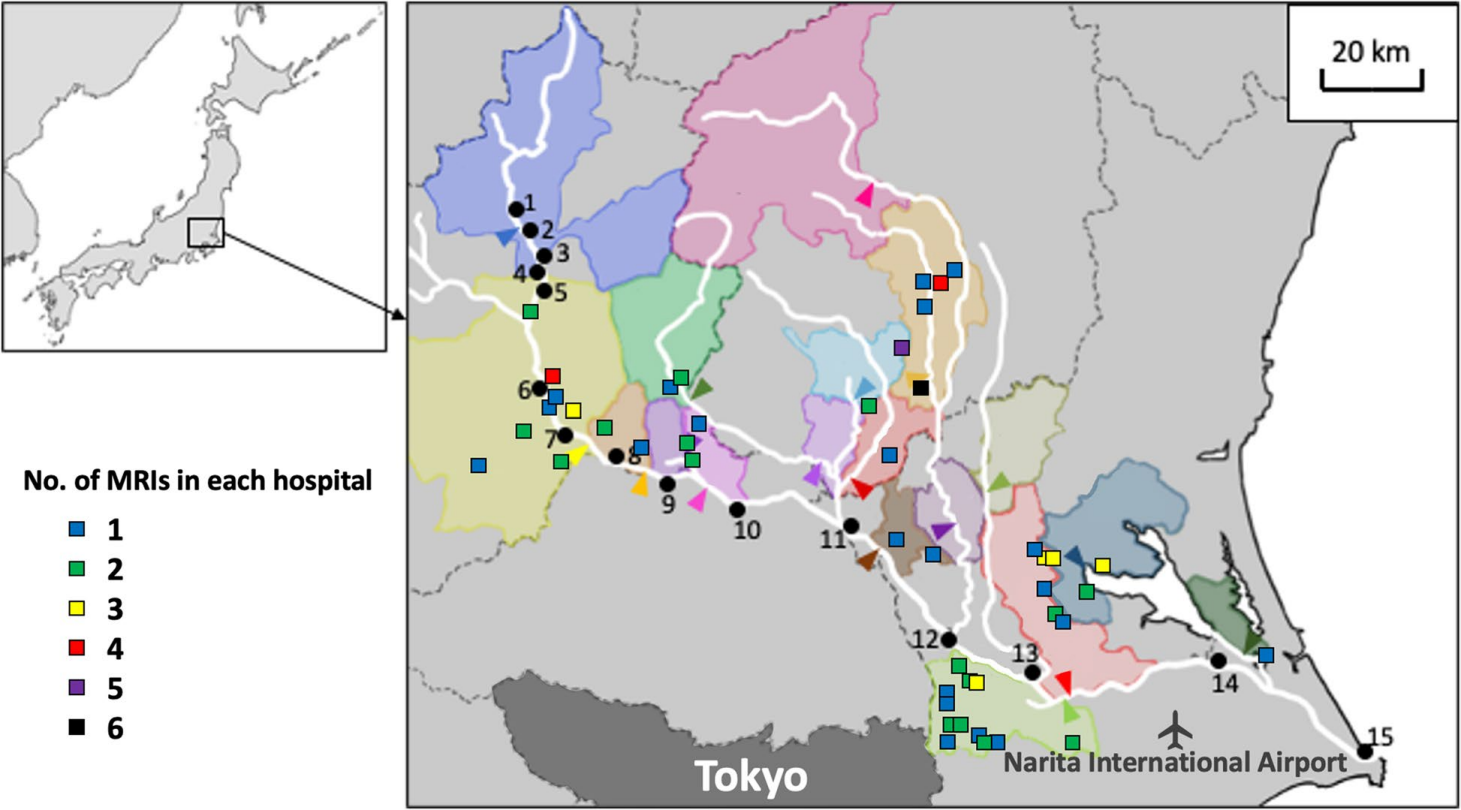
The location of major hospitals in each wastewater treatment area. The number corresponds to the sample code in Table 2 and Figs. 3 and 4. The colored area indicates each wastewater treatment area while the arrowhead indicates the locations of each WWTP output. Each square symbol represents a general hospital with more than 300 beds. The color of the square symbols represents the number of magnetic resonance imaging units owned by each hospital
